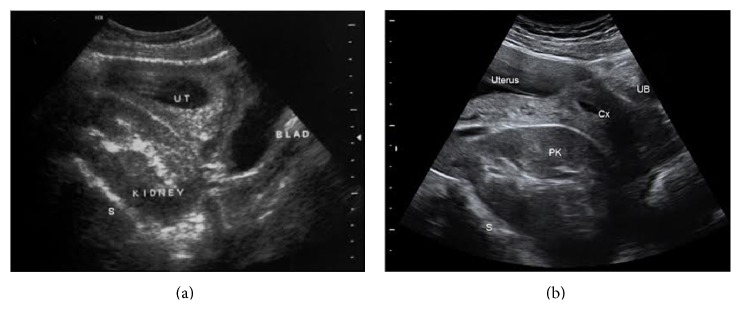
Transabdominal sonograms in the first and second trimesters. (a) shows a sagittal B-mode sonogram performed at the 12th gestational week. The pelvic kidney (PK) lies on the anterior sacral wall (S) below the uterus (UT) containing gestational sac. The inferoposterior wall of the urinary bladder (BLAD) touches the lower pole of the pelvic kidney. (b) demonstrates median plane sonogram performed at 23rd gestational week. Similar to (a) the pelvic kidney (PK) is deeply located in the maternal pelvis in front of the sacrum (S) and behind the uterine cervix (Cx). The uterus appears above the pelvic kidney. The empty urinary bladder is indicated by UB.
